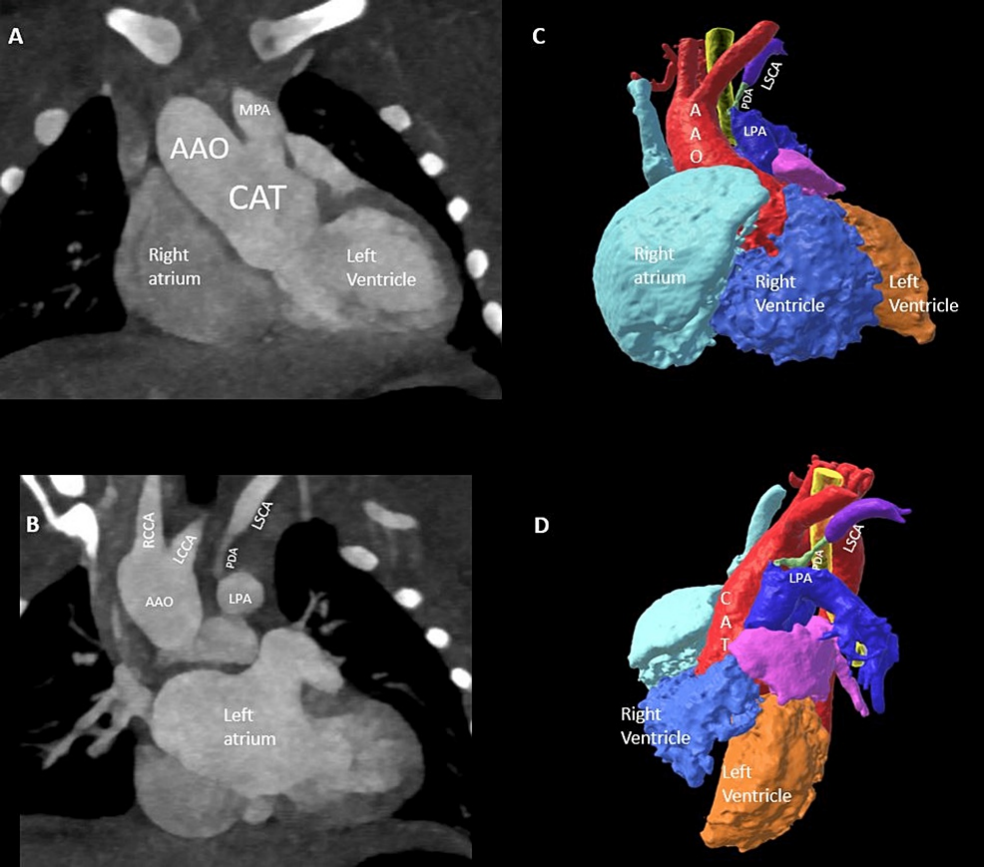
Truncus arteriosus with the ductal origin of the left subclavian artery. Coronal (A) reformatted CT angiogram image of the chest showing the type 1 truncus arteriosus. Reformatted coronal (B) CT image shows the origin of left subclavian artery (LSCA) from the ductus arteriosus (PDA), which, in turn, arises from the left pulmonary artery (LPA).  3D models frontal (C) and oblique (D) views show respective anatomy of truncus arteriosus with the ductal origin of left subclavian artery. CAT: Common arterial trunk, MPA: Main pulmonary artery, AAO: Ascending Aorta, LPA: Left pulmonary, artery, PDA: Patent ductus arteriosus, LSCA: Left subclavian artery, LCCA: Left common carotid artery, RCCA: Right common carotid artery.
